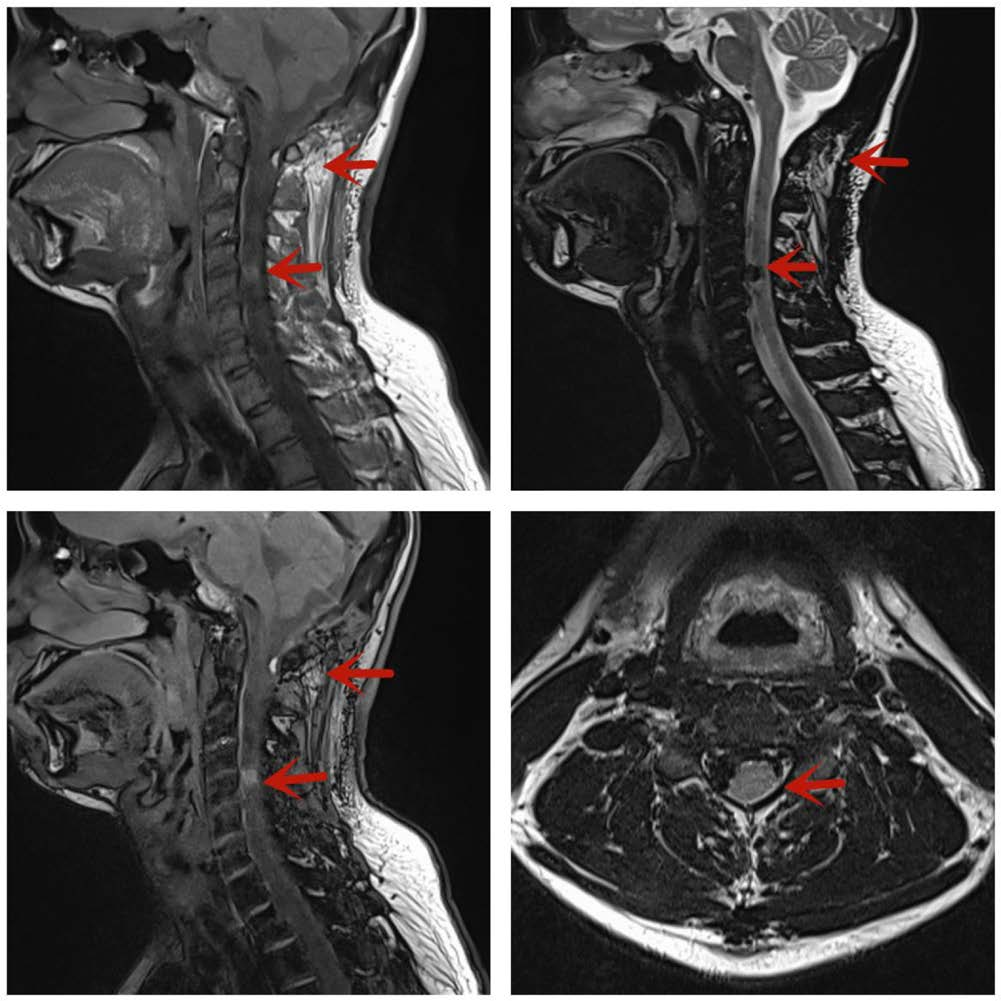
Magnetic resonance imaging (MRI) of the cervical spine showed a 10 × 7 mm cystic extramedullary mass between the C4 and C5 level, which was hyperintensity on T1-weighted imaging (T1WI), hypo-isointensity on T2WI and homogeneously enhanced with gadolinium diethylpentoic acid.
